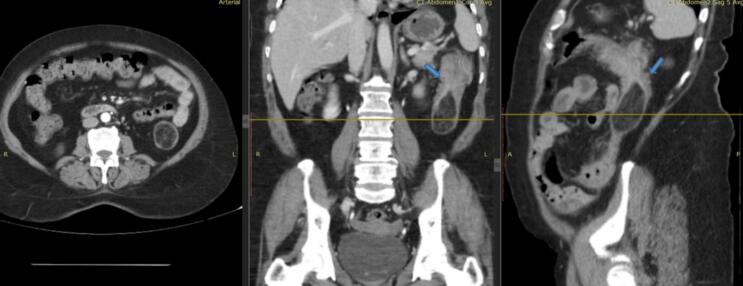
Contrast-enhanced abdominal CT images (axial, coronal, sagittal) showing incomplete intussusception of the descending colon with a well-circumscribed intraluminal fat-density mass (pedunculated lipoma) acting as the lead point (arrows).
